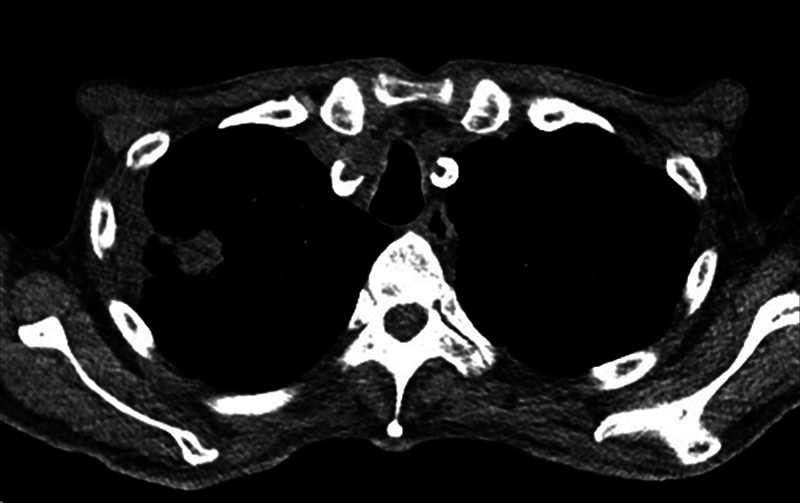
Computed tomography scans revealed a spiculated mass with infected bullae measuring 3.3 cm in the right upper lobe, with reports suggesting possible malignancy.
